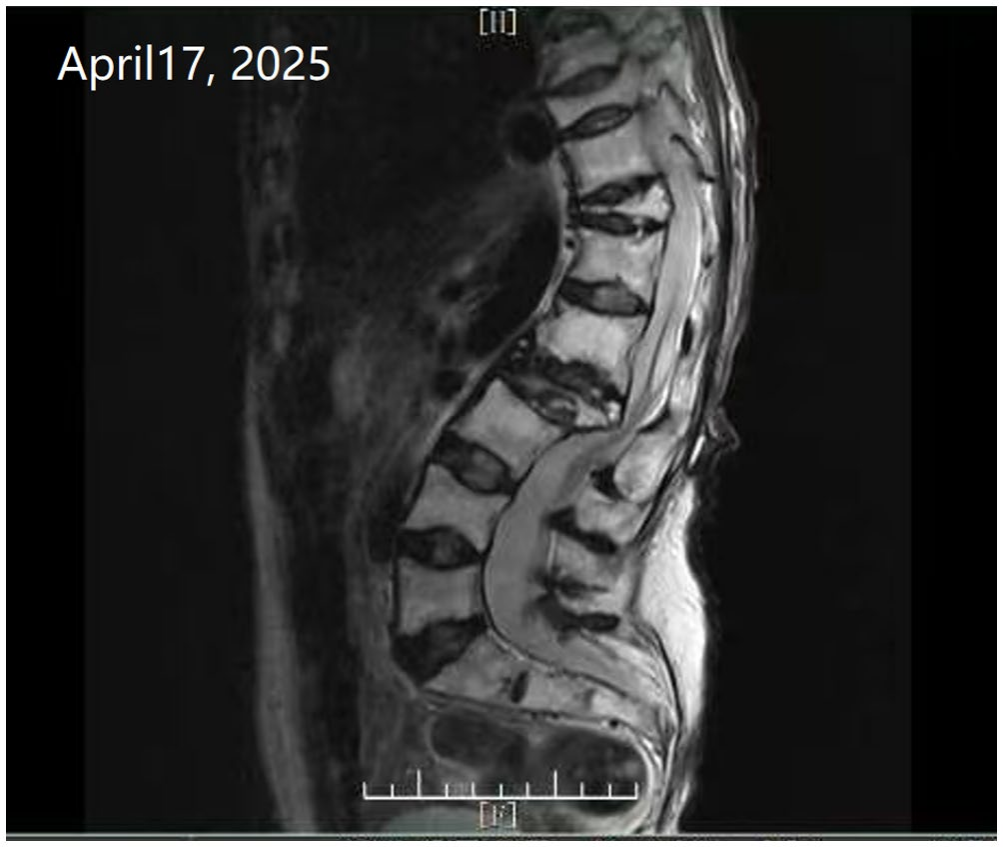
Inspection results: MRI: Lumbar degenerative changes, compression fractures of T11 and L2 vertebral bodies, disc herniation at the L1-S1 level. Spinal Surgery Consultation Findings: Change dressings regularly; If physical condition permits, surgical intervention is recommended; Actively treat the underlying disease.	After consulting multiple hospitals regarding the patient’s current condition, it has been determined that the prerequisites for surgery are not met. Follow-up plan: As the patient’s condition does not currently permit surgery, continue with foam dressing decompression and exudate collection. Avoid puncturing the hematoma for blood collection at this time (to prevent active bleeding).
